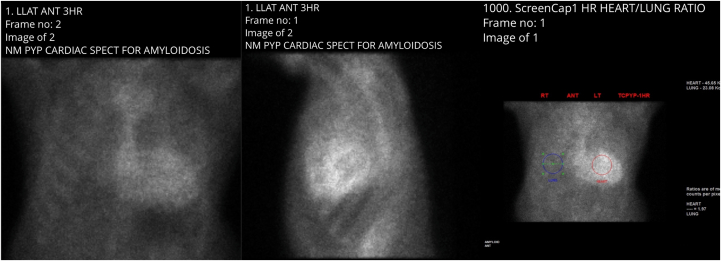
PYP Scan From left to right: 180-minute anterior view, 180-minute left lateral view, and heart-to-lung (H/CL) ratio. Diffuse myocardial retention of tracer is noted on the anterior view. H/CL ratio is 1.92 (normal ≤1.5). Single-photon emission computed tomography (SPECT) demonstrates marked diffuse uptake within the myocardium. PYP = technetium-99m pyrophosphate.
